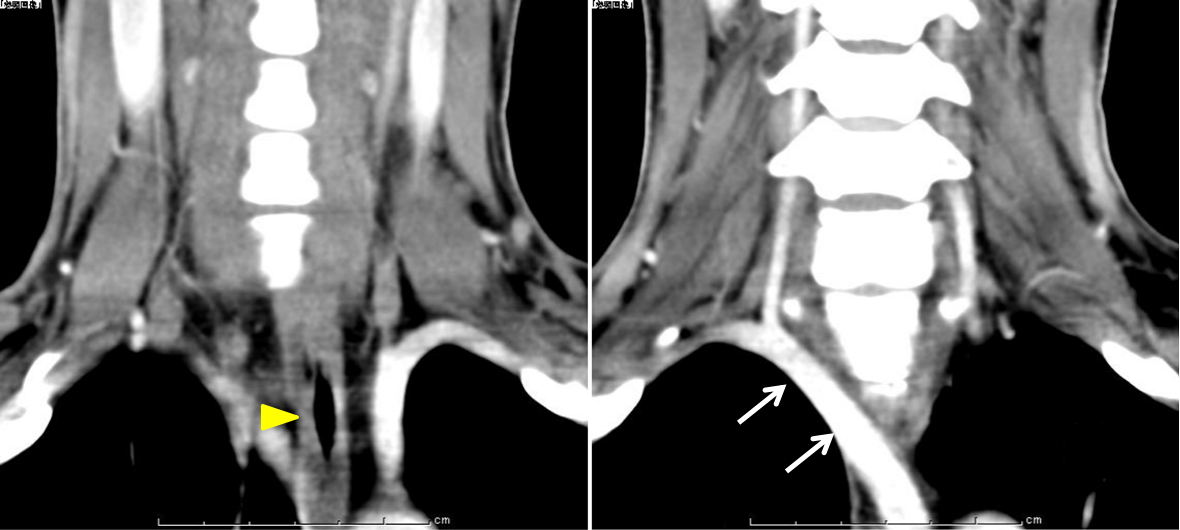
Preoperative enhanced CT scan of neck and chest of case 1. A right subclavian artery (*white arrow*) was found to originate from the aorta left of the midline and ran to the right axilla region crossing behind the esophagus (*yellow arrowhead*)
